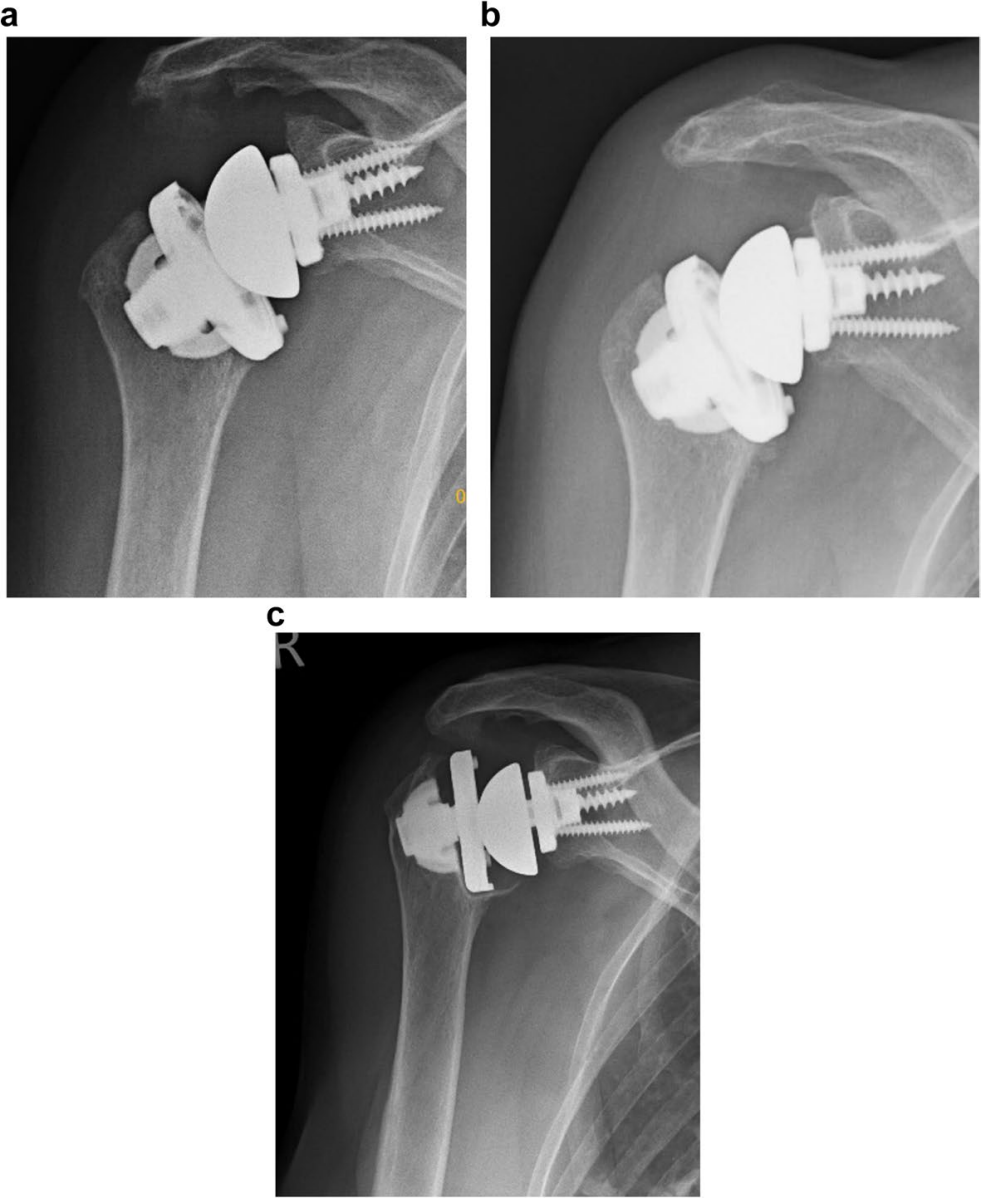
Postoperative X-ray imaging (a) and slight displacement of the Nano anchor 6 months after surgery (b). Severe atraumatic displacement, subsidence and peg breakage 7 years after surgery (c)
